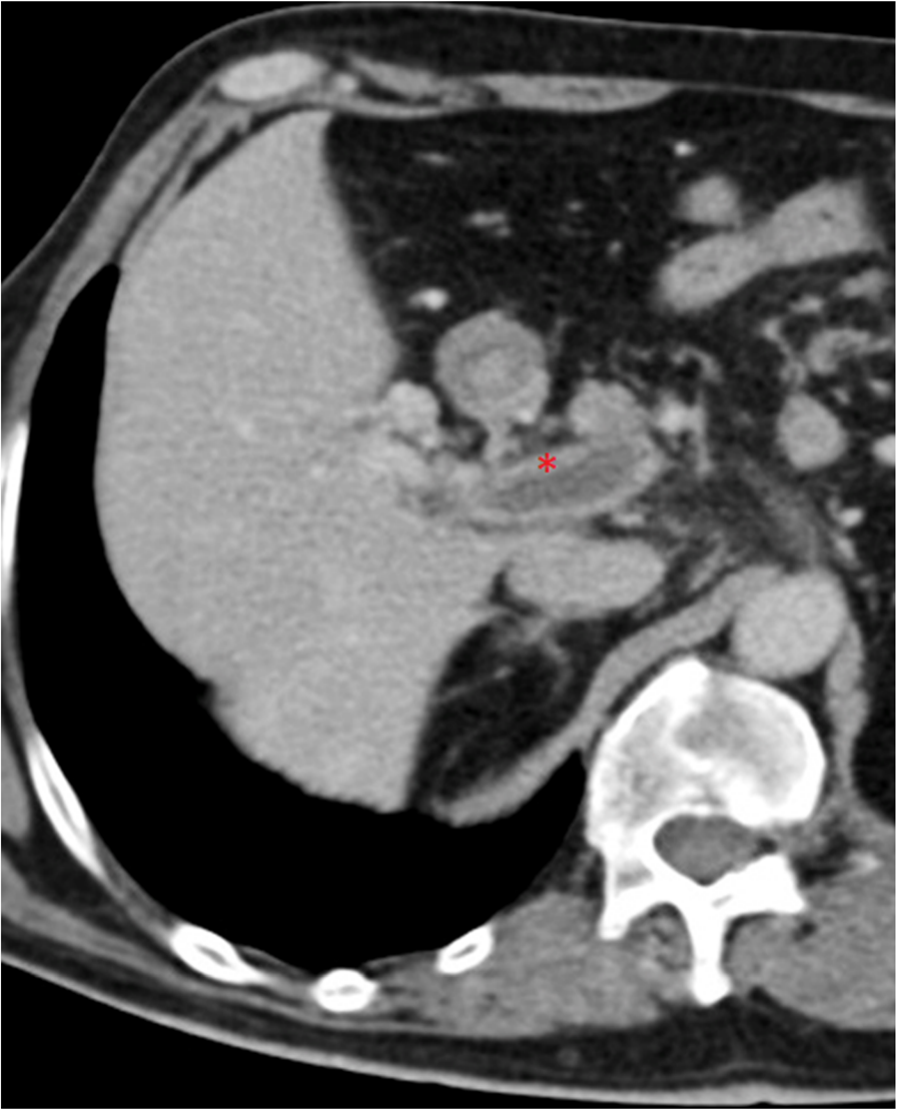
Acute portal vein thrombosis. MPR image from portal venous phase CT shows a luminal thrombus within the main PV, and enhancement of the vessel wall (red asterisk), presumably due to still normal flow through its *vasa vasorum*
